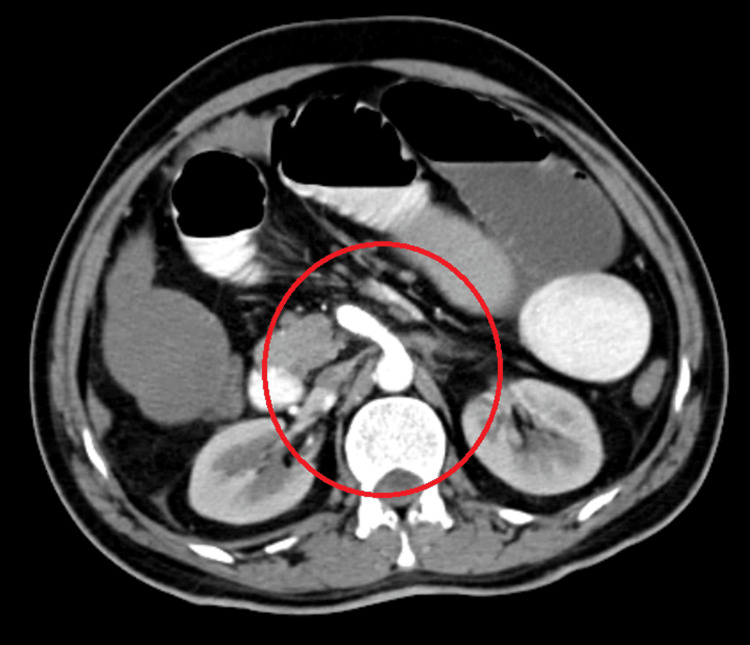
CECT abdomen pelvis: showing the stent in situ placed secondary to SMA occlusion CECT: contrast-enhanced computed tomography SMA: superior mesenteric artery
